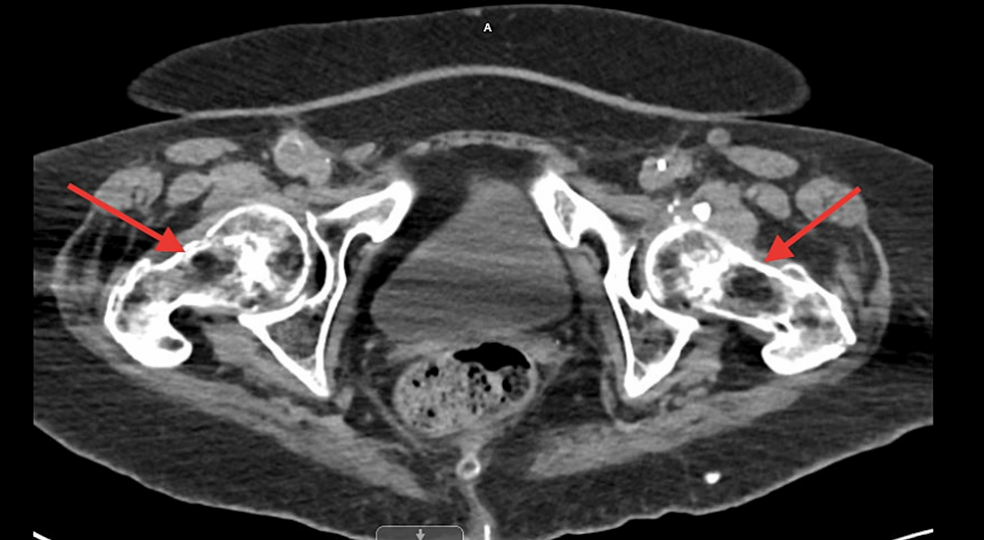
A CT angiogram of the abdomen showing heterogeneous masses in both proximal femurs, consistent with bony metastases
